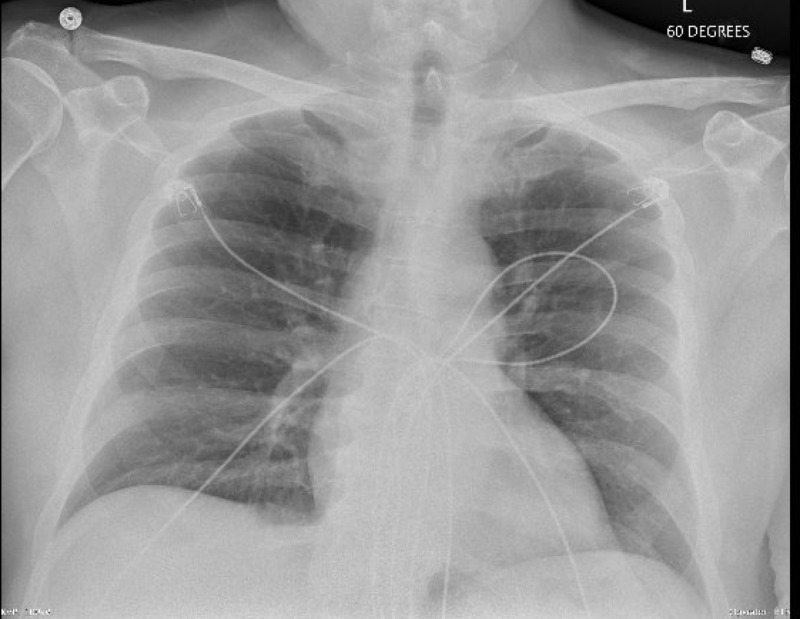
Chest X-ray. Posterior anterior view of the X-ray of the chest showing no features of acute infection
